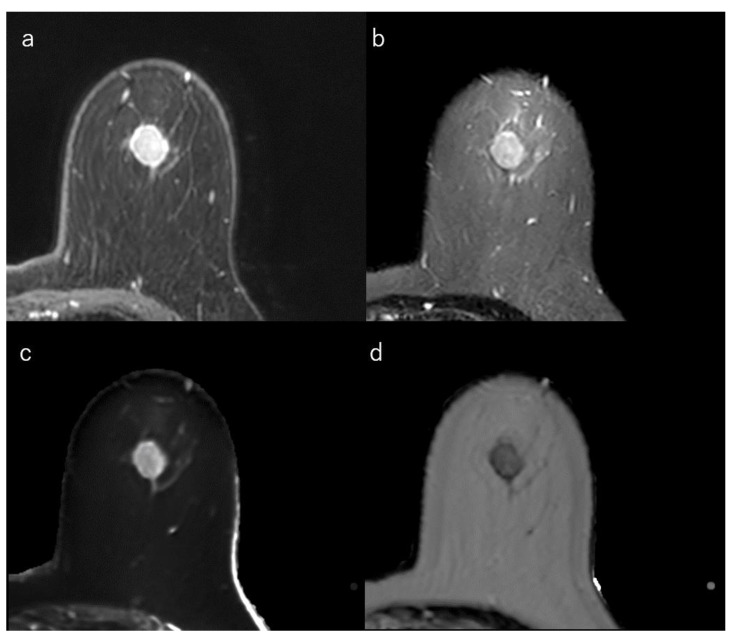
(a) Dynamic contrast-enhanced MR image in a 56-year-old woman with human epidermal growth factor receptor 2-enriched breast cancer shows a round mass. (b) Short-tau inversion recovery image shows a high signal mass. (c) T1 map (window width/centre = 1400/2400 ms) shows a high signal mass; mean T1 of the mass is 1256 ms, (d) T2 map (window width/centre = 160/240 ms) shows an intermediate signal mass; and mean T2 of the mass is 88 ms.
